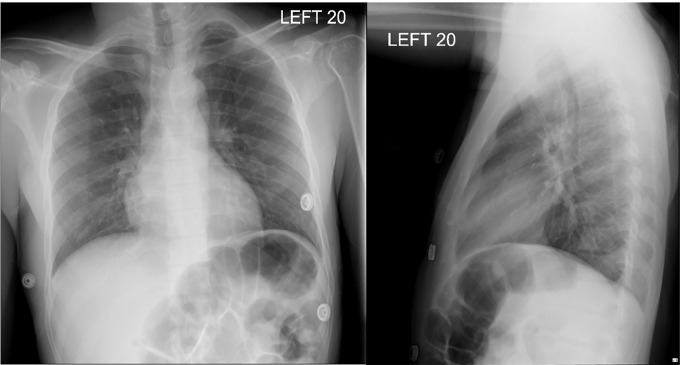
Chest radiograph posterior-anterior (left) and lateral (right) of a 36-year-old male with syncope.
